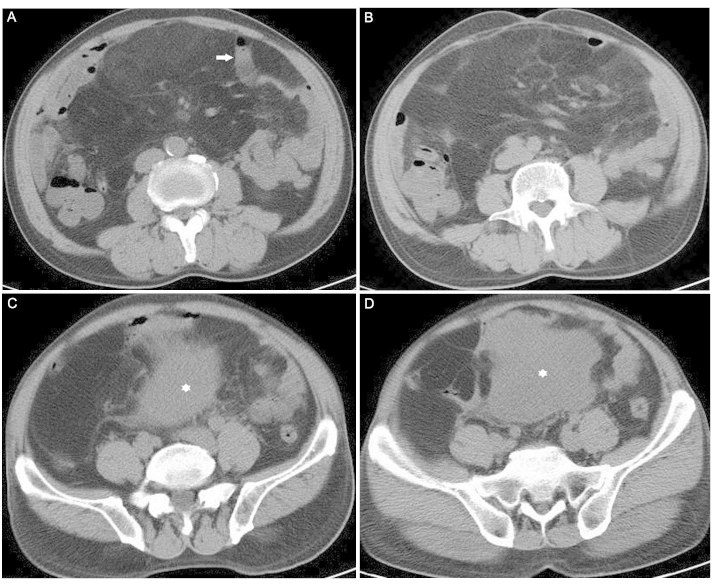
Unenhanced transverse computed tomography images of non-consecutive slices revealing a large, non-encapsulated abdominal-pelvic mass, with predominant fat attenuation. (A and B) The mass demonstrated a heterogeneous texture with coarse septa. A small bowel segment was entrapped in the fatty tissue (A; arrow). (C and D) The tumor also exhibited an inferiorly located area of globular non-adipose tissue, which was partially ill-defined (stars). Small bowel loops were displaced peripherally.
